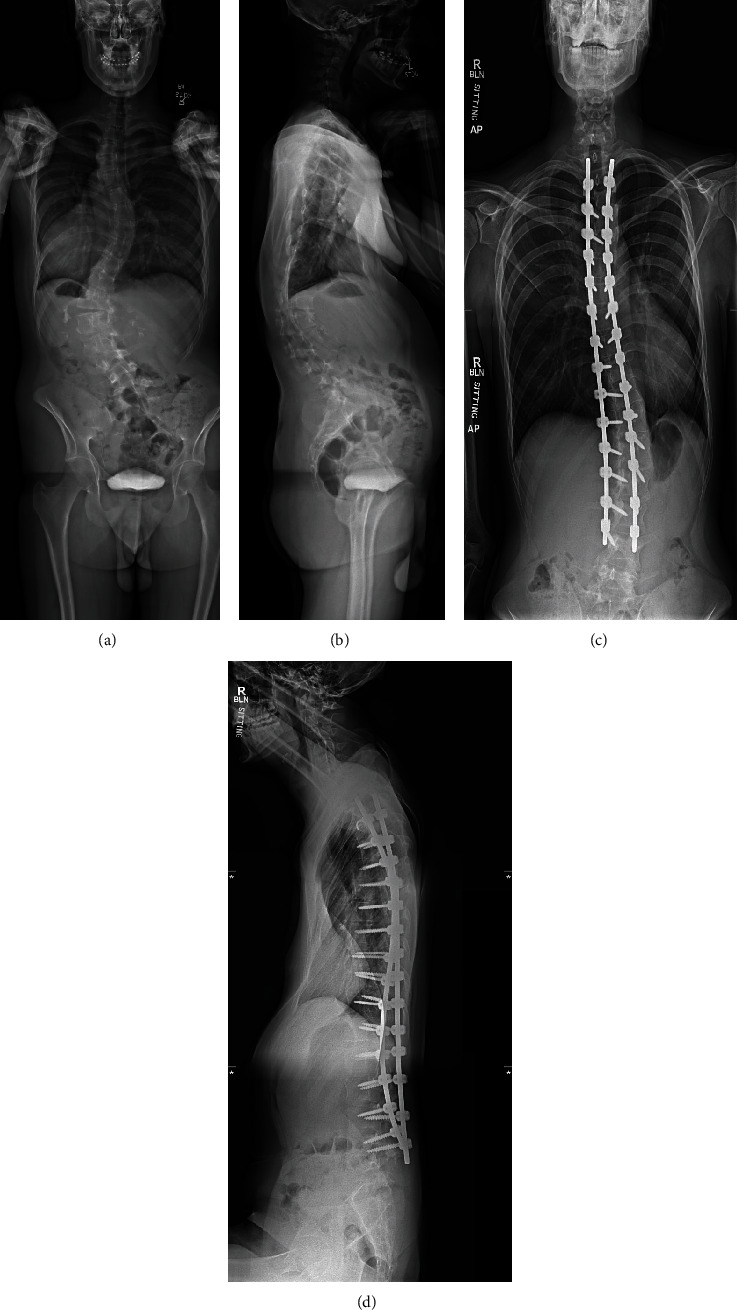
Preoperative and postoperative radiographs. The top two images are the preoperative standing posterior-anterior (a) and lateral (b) plain radiographs of the entire spine. The bottom two images are six-month postoperative standing anterior-posterior (c) and lateral (d) plain radiographs of the spine.
